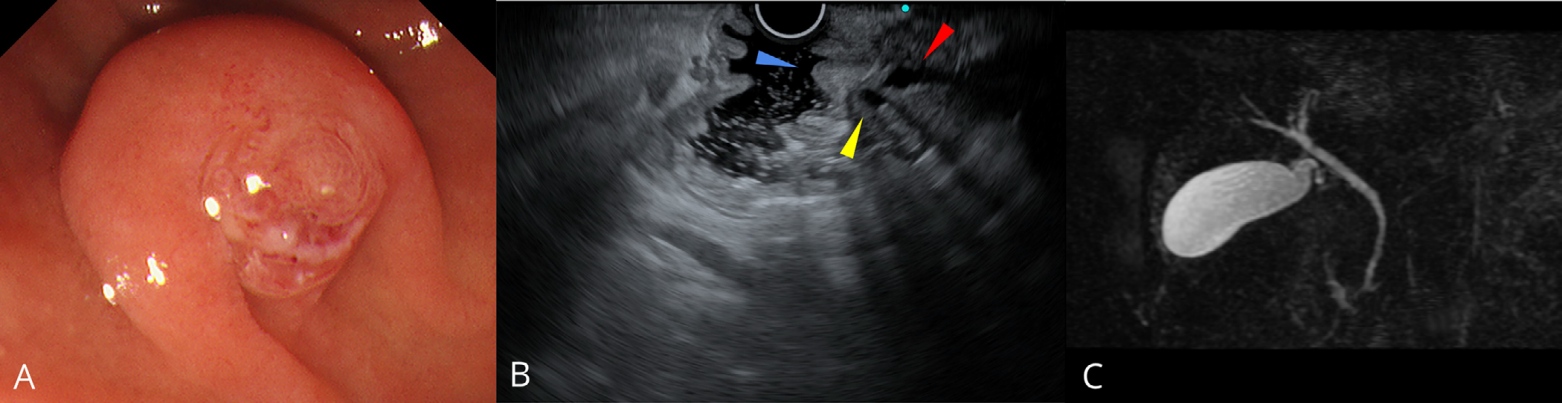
(A) Follow‐up esophagogastroduodenoscopy showing scarring at the biopsy site of the ampulla of Vater on day 4. (B) Endoscopic ultrasonography revealing no obvious abnormalities in the bile duct (red arrowhead), pancreatic duct (yellow arrowhead), or ampulla of Vater (blue arrowhead). (C) Magnetic resonance cholangiopancreatography revealing no abnormalities in the bile or pancreatic ducts.
